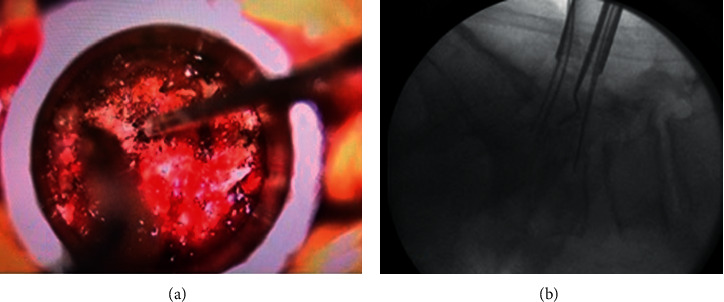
Grade 1 L5-S1 spondylolisthesis with the bilateral pars defect. (a) Intraoperative visualization of left L5 pars fracture (horizontally oriented). (b) X-ray confirmation of the pars fracture location (after placement of pedicles, *K* wires, and tubular exposure).
